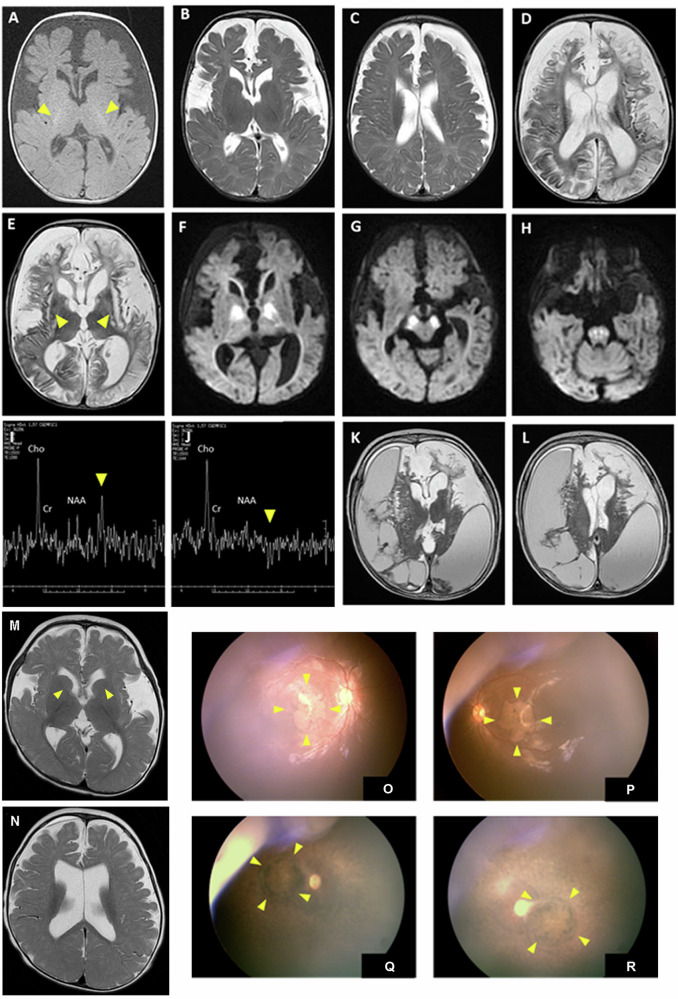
Brain imaging and ophthalmological findings in PAICS-deficient patients. A–C Brain image changes in patient 1 axial MRI at 3 months of age. Axial T1-weighted MRI image A revealed visible myelination of only the posterior limb of the internal capsules (arrowheads), enlargement of the ventricles, chronic subdural effusion predominantly in the frontotemporal region, and widened Sylvian fissures with failed operculization. These features were also evident in axial T2-weighted imaging B, C. D–J
